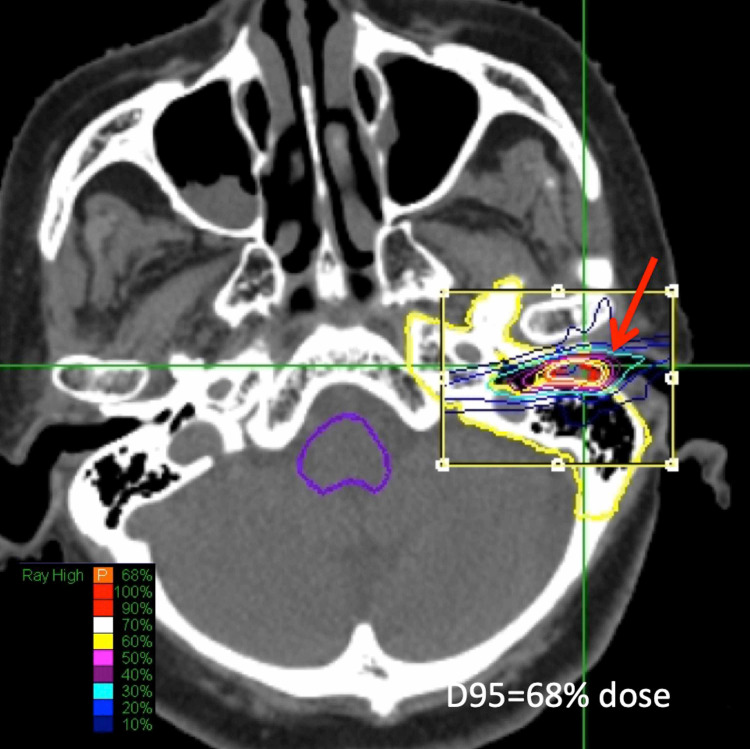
Dose Planning for Case 1 Axial image of iodine enhancement computed tomography (CT) on CyberKnife MultiPlan (Accuray, Tokyo, Japan) workstation showed excellent conformity for a small external auditory canal tumor (arrow). A prescription dose of D95%=24 Gy/ 3 fractions was adopted. After treatment, complete remission was obtained and maintained for 9 years.
